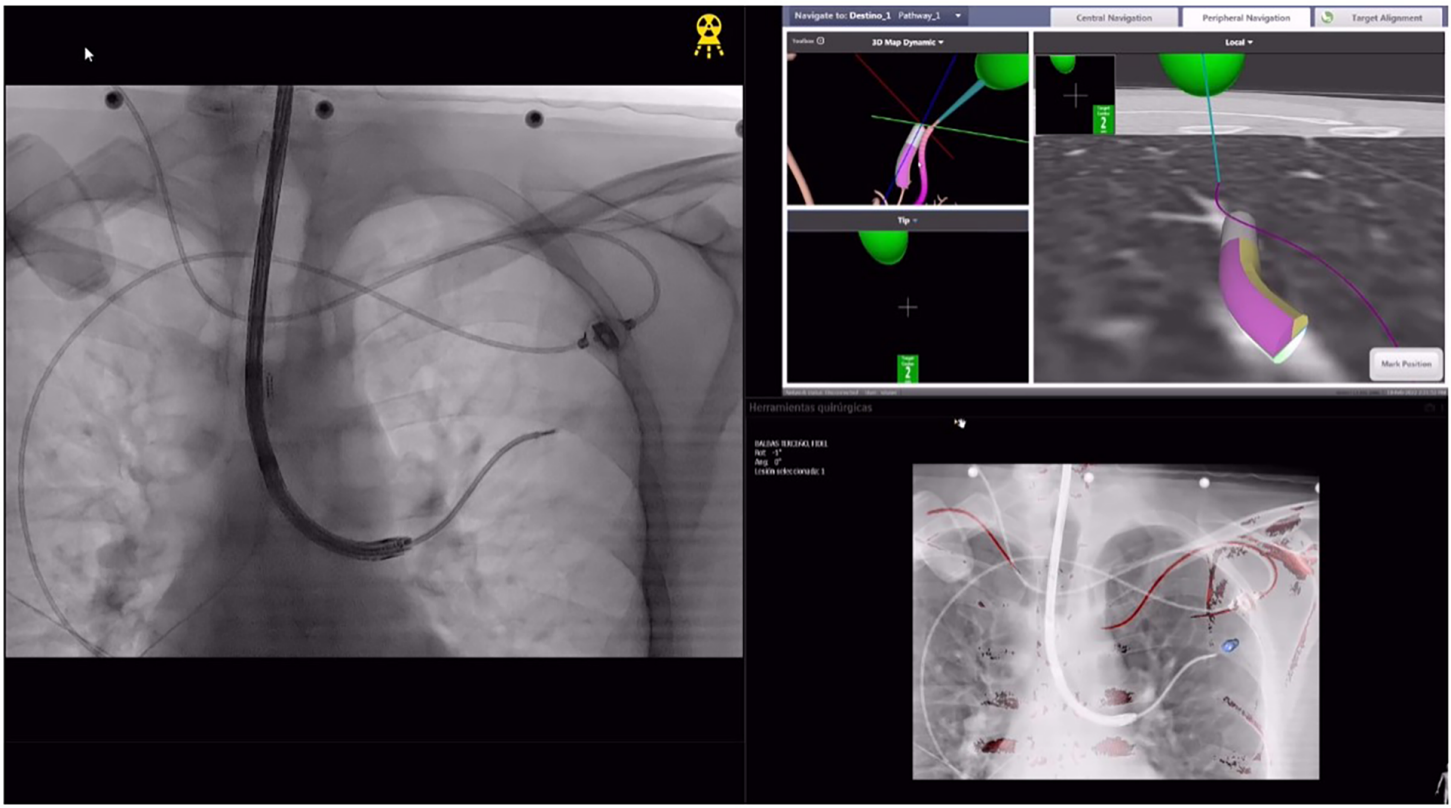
Intraoperative view of indocyanine green (ICG) marking performed with electromagnetic navigation bronchoscopy (ENB) and cone beam computed tomography (CBCT) assistance. This image is linked to Figure 1 and depicts the same case.
